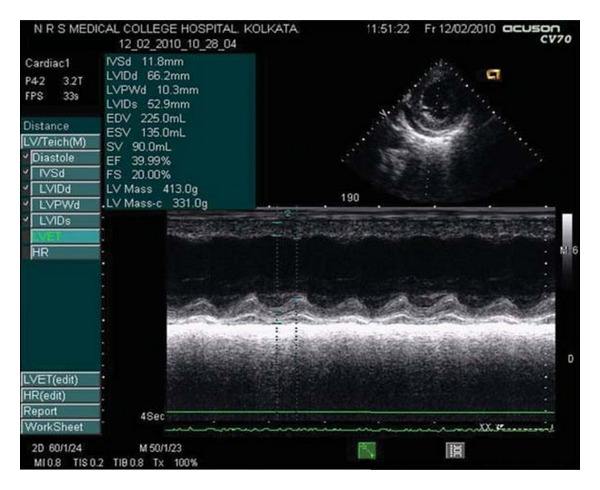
Pretreatment echocardiography showing severely depressed left ventricular systolic function.
